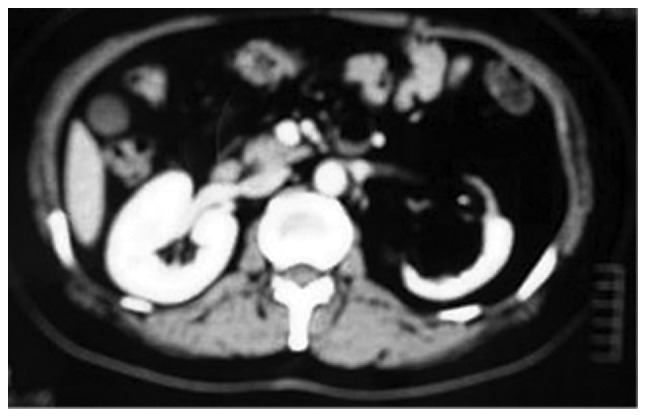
Case 3. Contrast-enhanced computed tomography (CT) scan of the abdomen showed a non-enhancing, homogenous and obvious mass measuring 8×5×5 cm in the left renal sinus with a CT value ranging from −50 HU to −35 HU.
